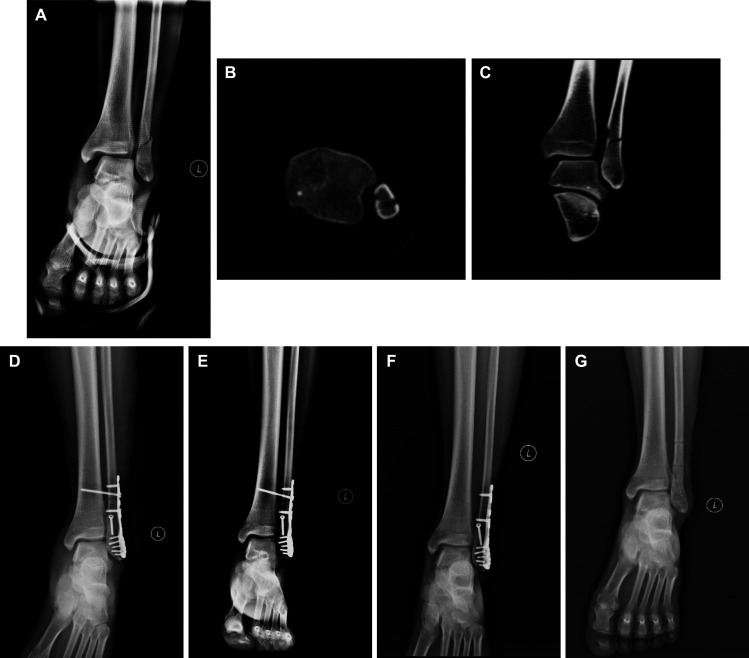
A Male patient with Weber type B, Lauge–Hansen SER-III, bimalleolar equivalent fracture treated with fibula ORIF and trans-syndesmotic screw fixation. Preoperative anteroposterior x-ray image (A), axial CT scan image (B), and coronal CT (C) scan image showed the fibular fracture, increased medial malleolus clear space and inferior tibiofibular syndesmotic disruption. (D) Postoperative anteroposterior image showed the reduction of fibular fracture, medial malleolus clear space, and distal tibiofibular joint. One month (E), one year (F), and post internal fixation removal (G) anteroposterior x-ray images did not show any displacement or dislocation, no ankle osteoarthritis was found either. ORIF, open reduction and internal fixation; CT, computed tomography.
